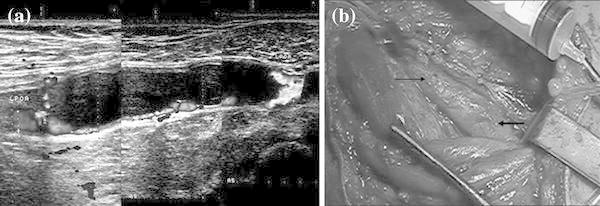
Case 2: a 36-year-old man with CAD of the popliteal artery underwent surgical excision of the cyst and interposition prosthetic bypass grafting. a Color Doppler ultrasound showed a cystic mass posterior to the popliteal artery causing endoluminal stenosis. Only a slim blood signal was observed between the cystic mass and the popliteal anterior wall. b Intraoperatively, the involved popliteal artery exhibited a cystic protuberance (*thin arrowhead*) with a normal proximal arterial lumen (*thick arrowhead*)
